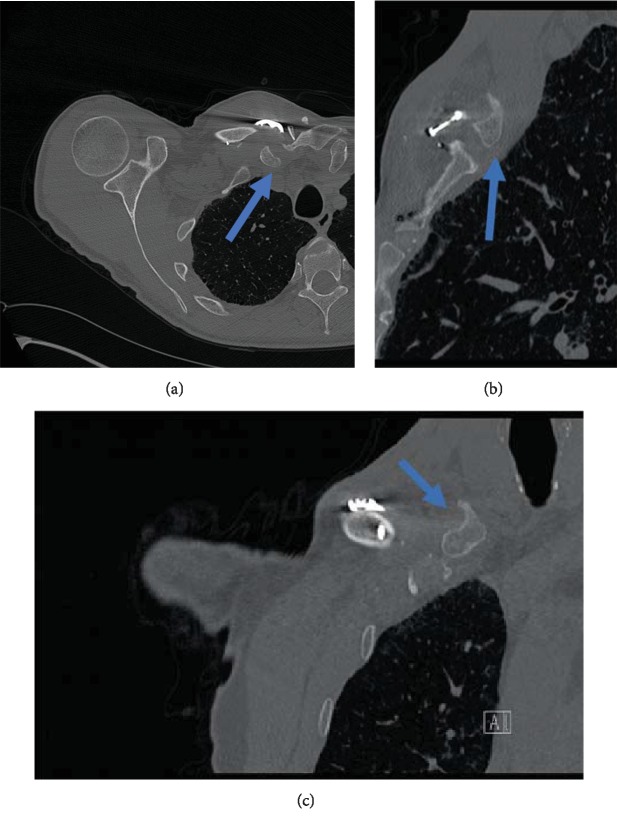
Five months post-ORIF. CT images showing hardware migration and posterior displacement of the remaining medial end of the right clavicle (blue arrow) (a–c).
